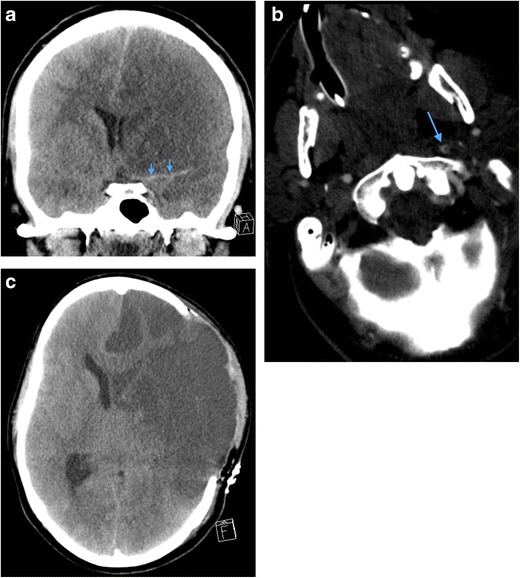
a Coronal reformatted non-contrast CT brain image of a 34-year-old man who sustained a penetrating knife injury to the left zone 2 of the neck requiring emergency surgical neck exploration prior to imaging assessment because of catastrophic haemorrhage. Therefore, pre-treatment CT and MDCTA were not performed because of the clinically evident active life-threatening haemorrhage on arrival in the emergency department. The non-contrast CT brain assessment was performed immediately prior to the post-operative MDCTA (b) and demonstrates acute thromboembolic “malignant” left anterior circulation infarction: there is already ischaemic demarcation of both the left middle and anterior cerebral artery territories with severe subfalcine herniation and midline shift; hyperdense thromboembolus is evident in the left middle cerebral artery (*arrows*) and proximal left anterior cerebral artery. b Axial image of MDCTA of the same patient as in a performed immediately post-operatively following internal carotid artery repair reveals a tail of extensive intraluminal thrombus extending distally from the site of vessel injury and repair (*arrow*). c Axial non-contrast CT brain of the same patient as in a and b performed 48 h later. The patient has undergone interval emergency left decompressive hemicraniectomy: the left anterior circulation low attenuation infarction is more established and the significant mass effect, subfalcine herniation and intracranial pressure related to swollen oedematous parenchyma have been ameliorated by hemicraniectomy. Unfortunately, brain-stem death was confirmed 12 h later
